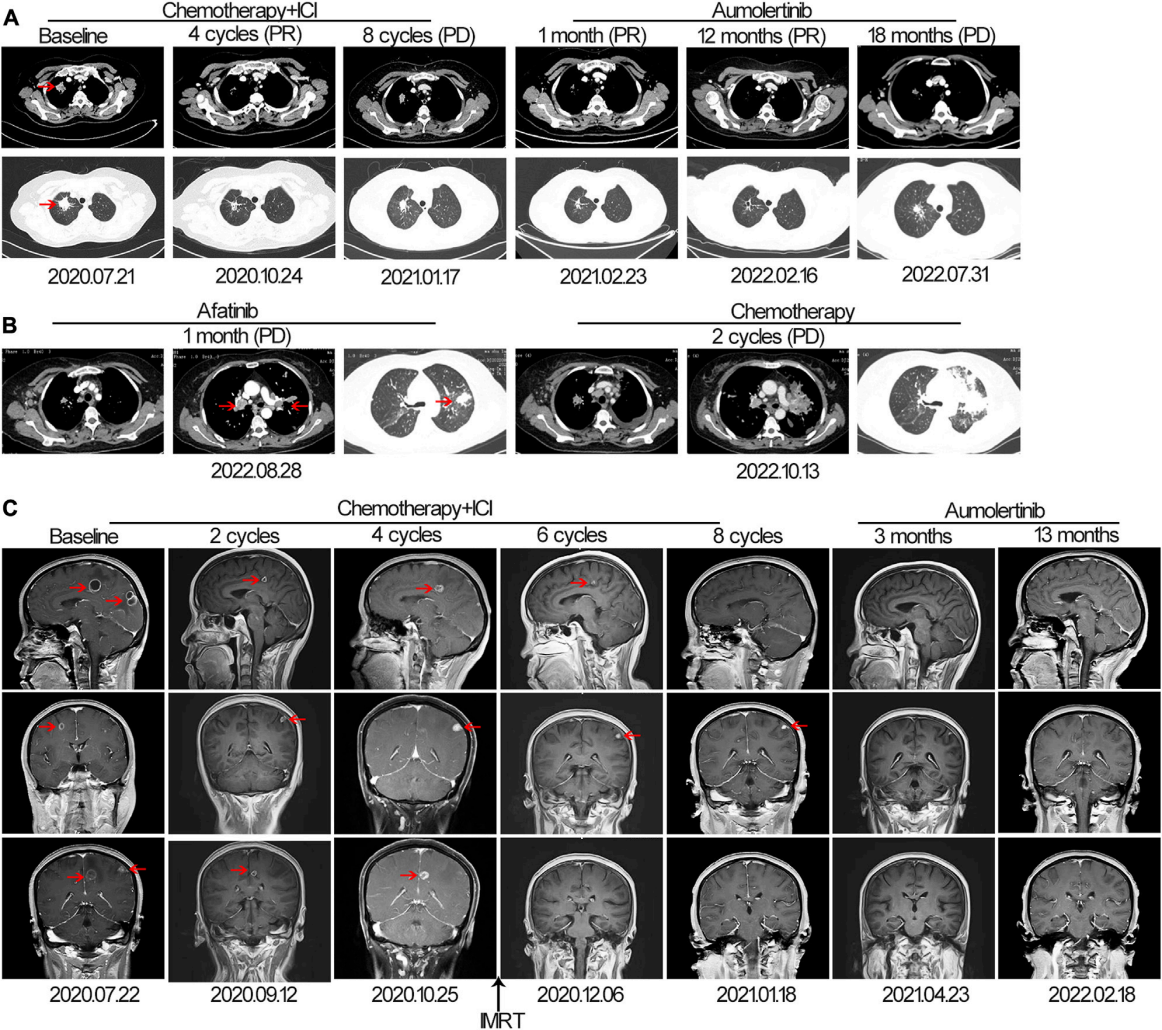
The tumor progression of the patient before and after treatment. (A, B) Representative lesions in the lung lobes according to computed tomography images at various points. (C) Magnetic resonance images at various points. The tumor is indicated by red arrows. ICI, immune checkpoint inhibitor; IMRT: intensity-modulated radiotherapy; PD, progressive disease; PR, partial response.
